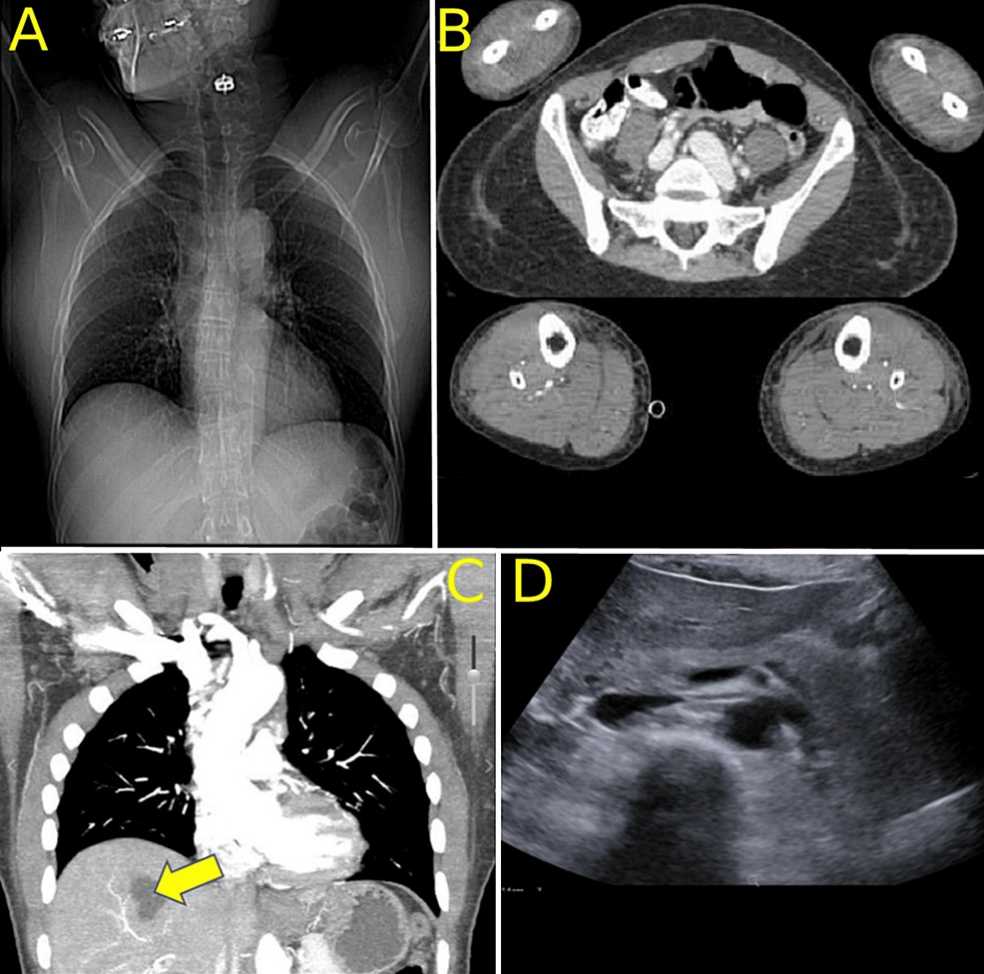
Initial Workup Imaging Results. Imaging obtained during her initial evaluation included: A) Anteroposterior radiograph obtained by CT, B) CTA of extremities (with runoff) in the axial plane to rule out vascular thromboses, C) CTA, in the coronal plane, which reported unknown “hepatic cysts,” with D) follow-up ultrasound of cysts, months later, showing a benign appearance. Additional imaging included vascular ultrasounds of all extremities to rule out thromboses, which were excluded due to the lack of information provided by a single frame. They were interpreted as showing nonspecific waveform abnormalities in the right upper extremity arterial Doppler US.
